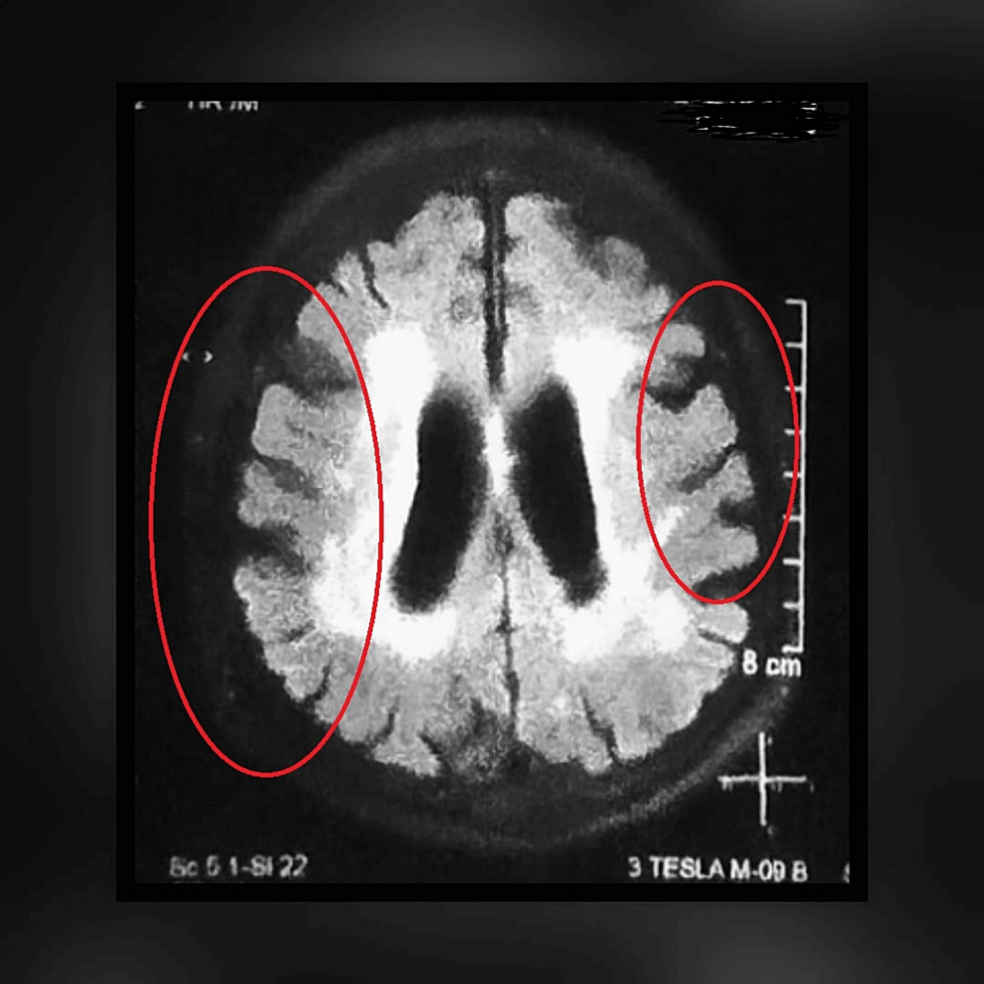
Magnetic resonance imaging (MRI) of brain showing cortical ribboning typical of Creutzfeldt Jakob disease.
